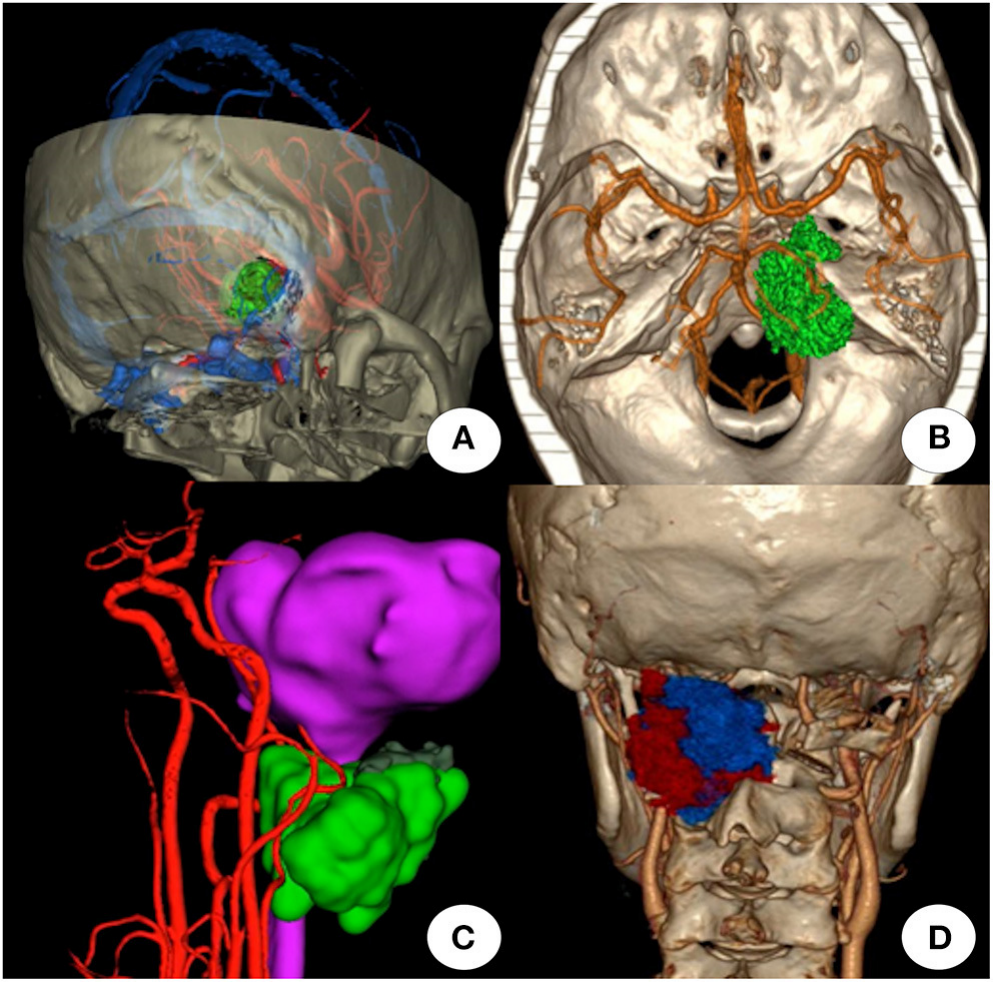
Effectiveness of multimodal image fusion technology. (A) Surgical plan of the retrosigmoid approach using a multimodal image fusion image (combined with head computed tomography, magnetic resonance imaging, magnetic resonance angiography, and magnetic resonance venography). (B) Multimodal image fusion demonstrates the spatial relationship of the tumor and surrounding structures. (C) Multimodal image fusion demonstrates the relationship between the left vertebral artery and tumor (green). (D) Multimodal image fusion demonstrates the spatial relationship between the tumor (red and blue), artery, and surrounding bony structures.
